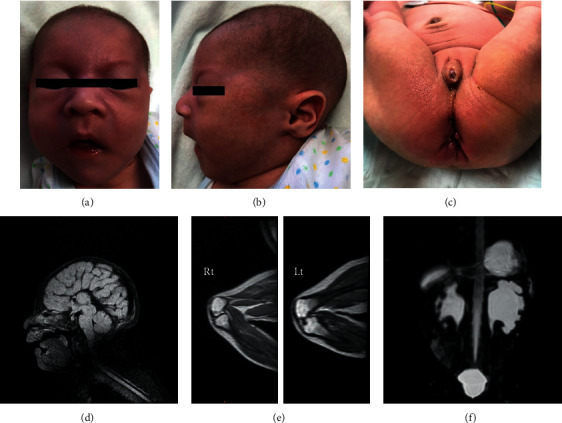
Photographs and MRI of the patient. (a) A front view of the patient showing bitemporal bulbous nose, wide nasal base, and bitemporal narrowing. (b) A side view of the patient showing microcephaly, micrognathia, and retrognathia. (c) Perineum showing hypoplastic scrotum. (d) A sagittal fluid-attenuated inversion recovery (FLAIR) image showing corpus callosum agenesis. (e) Sagittal T2-weighted MRI images of the knees showing absent patellar cartilages. (f) A coronal 3D T2-weighted image showing bilateral renal pelvic dilation.
